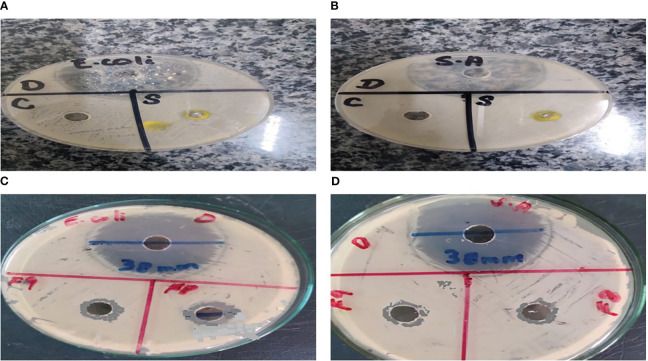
Antibacterial activity of *Carthamus tinctorius* L. (CT) oil against (A)
